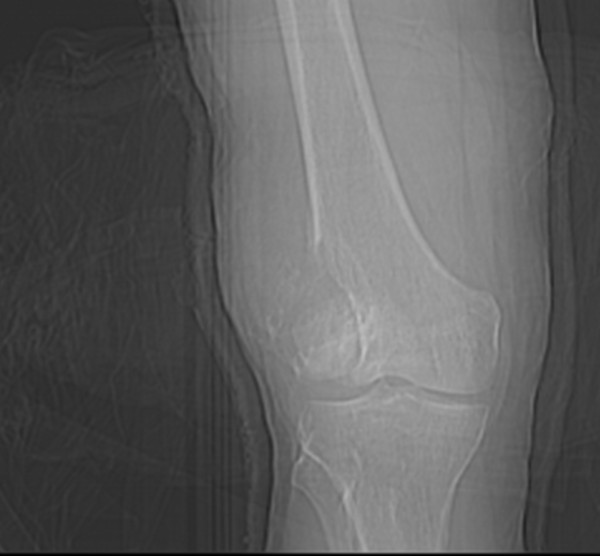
X-Ray lower end of femur showing Giant Cell Tumor.
